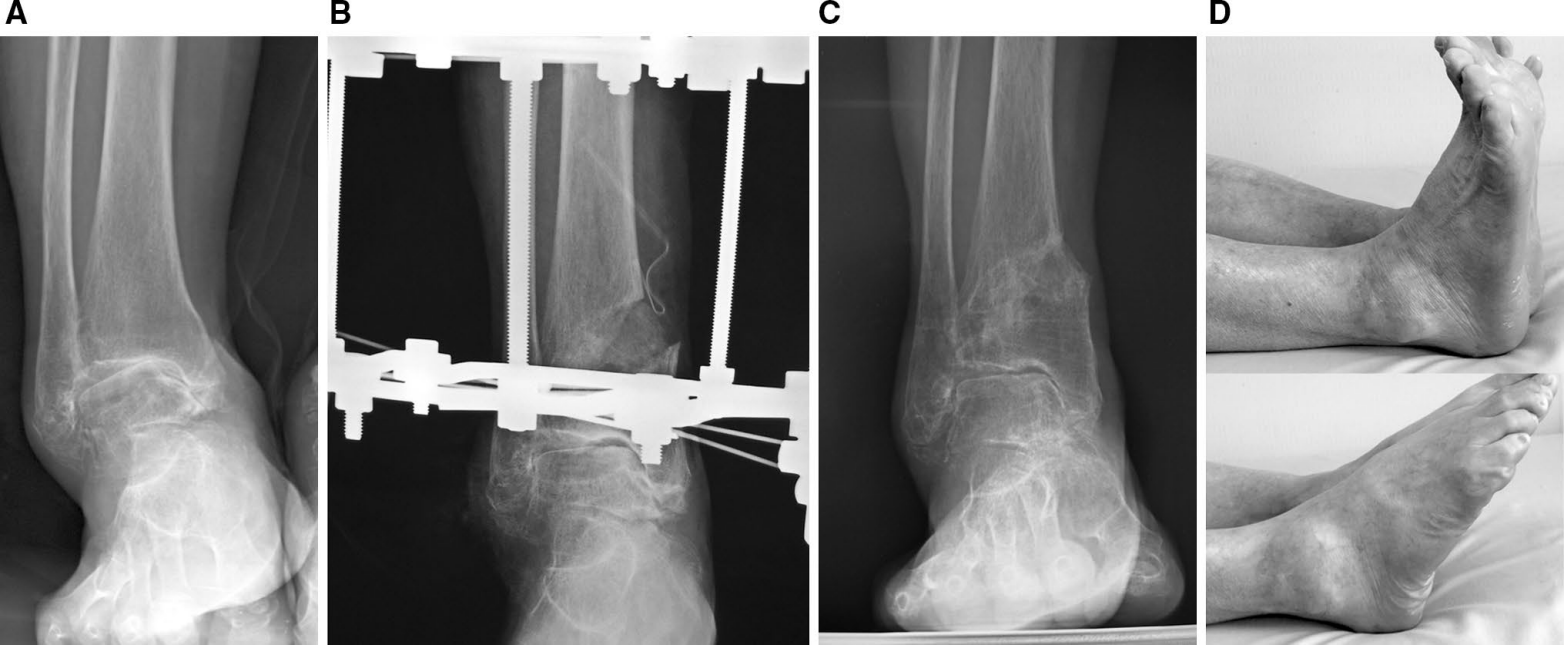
A 69-year-old woman with stage 3b varus osteoarthritis in her right ankle underwent a DTOO. The weight-bearing radiograph before surgery showed the severe varus deformity and joint space narrowing with obliteration of this joint space on the roof of the dome of the talus (a). During surgery, the dorsiflexion measured -10° after osteotomy; the Achilles tendon was elongated to overcome this equinus. Bony spurs present in the fibula and the tibia were removed owing to the impingement caused. The tibia and osteotomy was fixed by an Ilizarov external fixator (b). The instability of ankle joint before DTOO was improved with the talar articular surface and plantar surface of the hindfoot almost perpendicular to the tibial axis (b, c). The severe ankle joint pain was resolved completely. The range of motion was maintained 2 years after DTOO (dorsiflexion 0°; plantar flexion 30°) (d). The patient could return to farm work without pain
